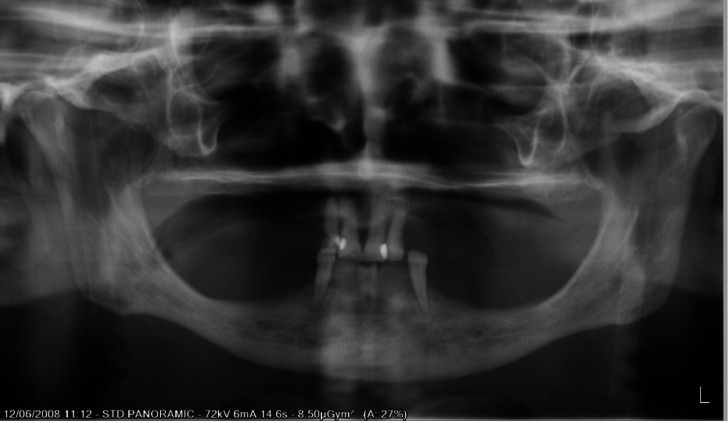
Orthopantomography of the patient on his first visit.
